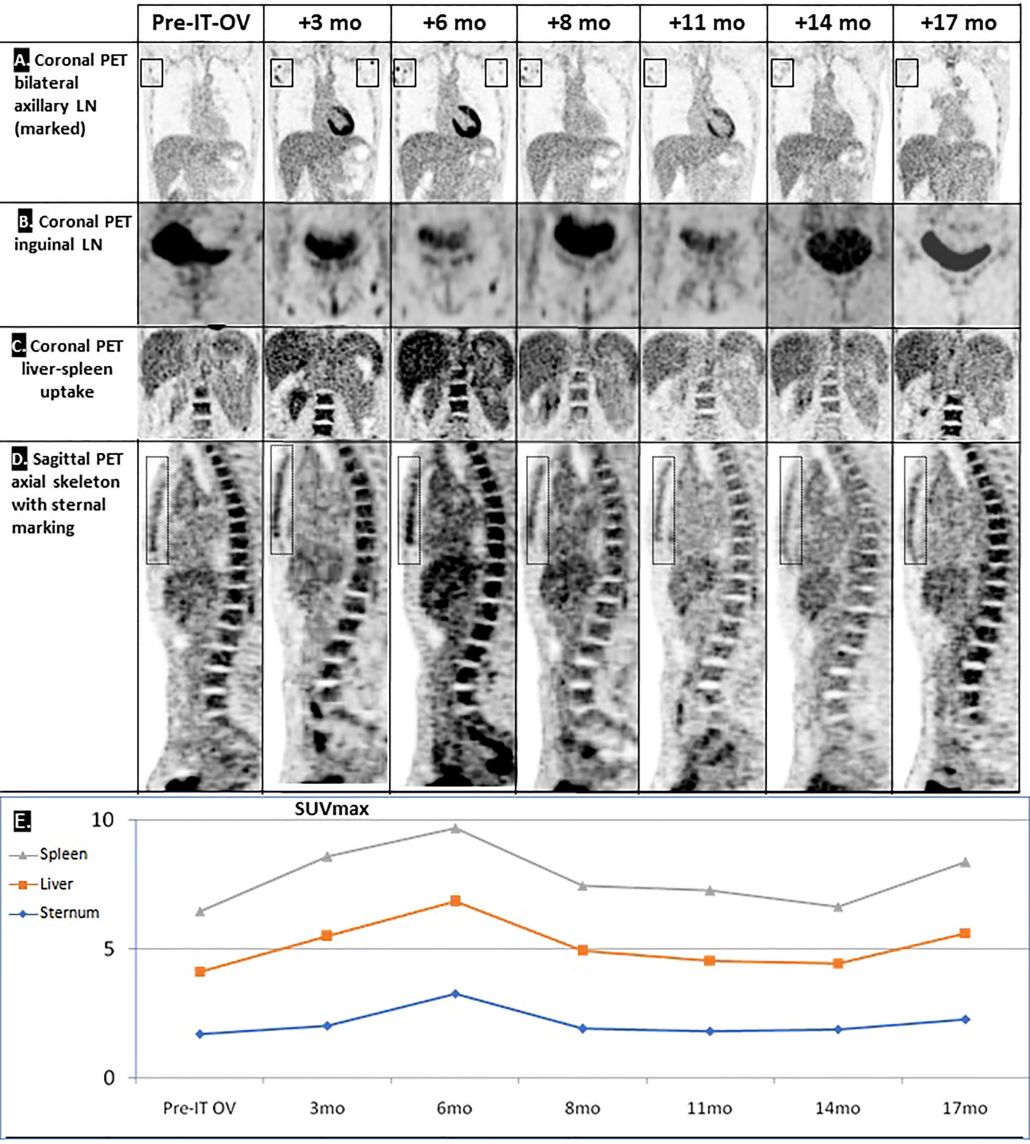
Hyperimmune reaction following IT-OV immunotherapy. Coronal PET showing an apparent OV-stimulated hyperimmune reaction in the local (A) and distant (B) lymph nodes (LN), in the liver-spleen (C), and in the axial skeleton (D). Graphic summary of chronological radiological pattern of the hyperimmune response, as measured by PET (SUV_max)_
